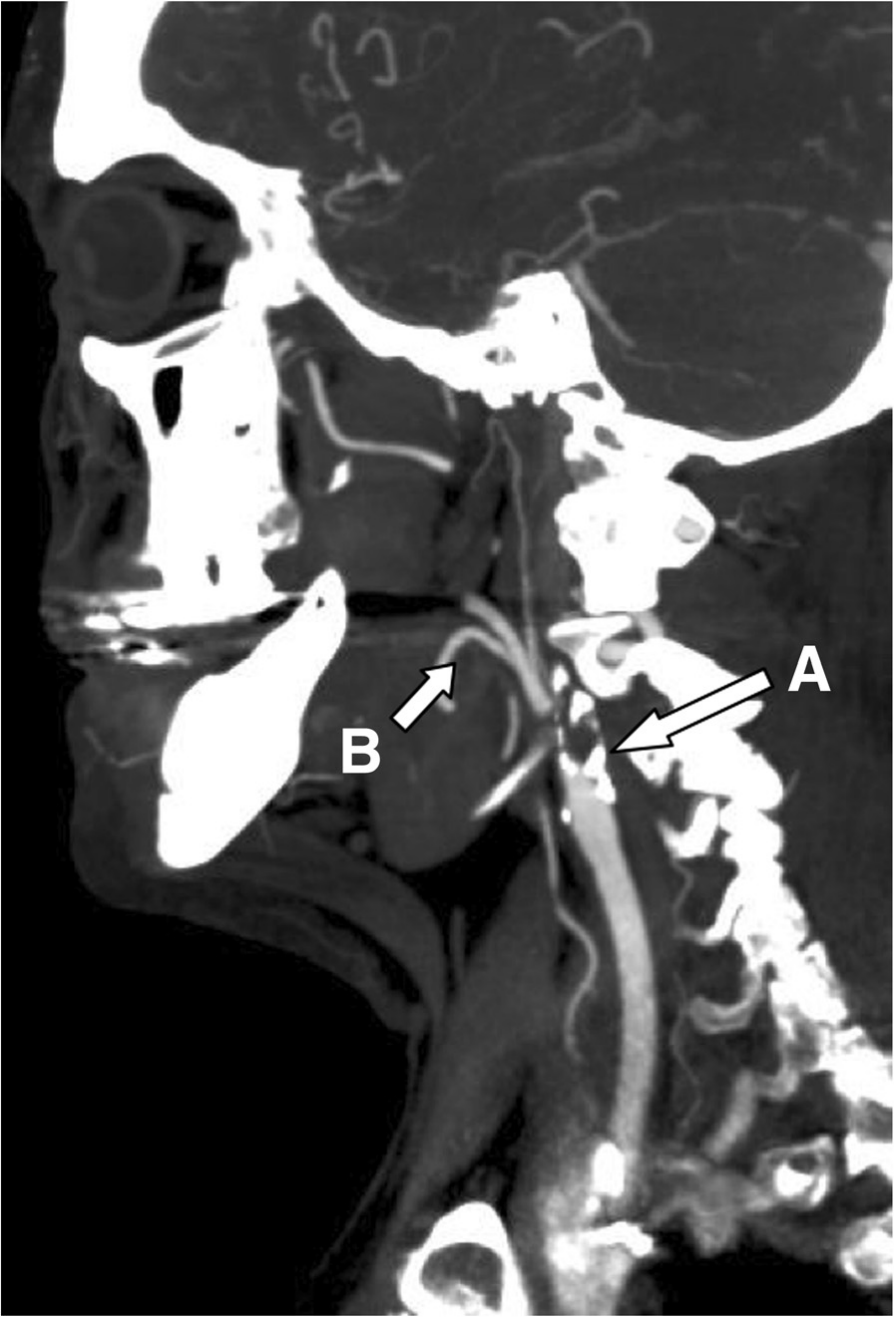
Initial CT-angiography showing left carotid occlusion. CT-angiography of the left carotid artery demonstrates calcified plaque of the left carotid bulb with atherosclerotic occlusion of the internal carotid artery (a). Of note the left external carotid artery is patent and there is robust filling of its branches (b)
